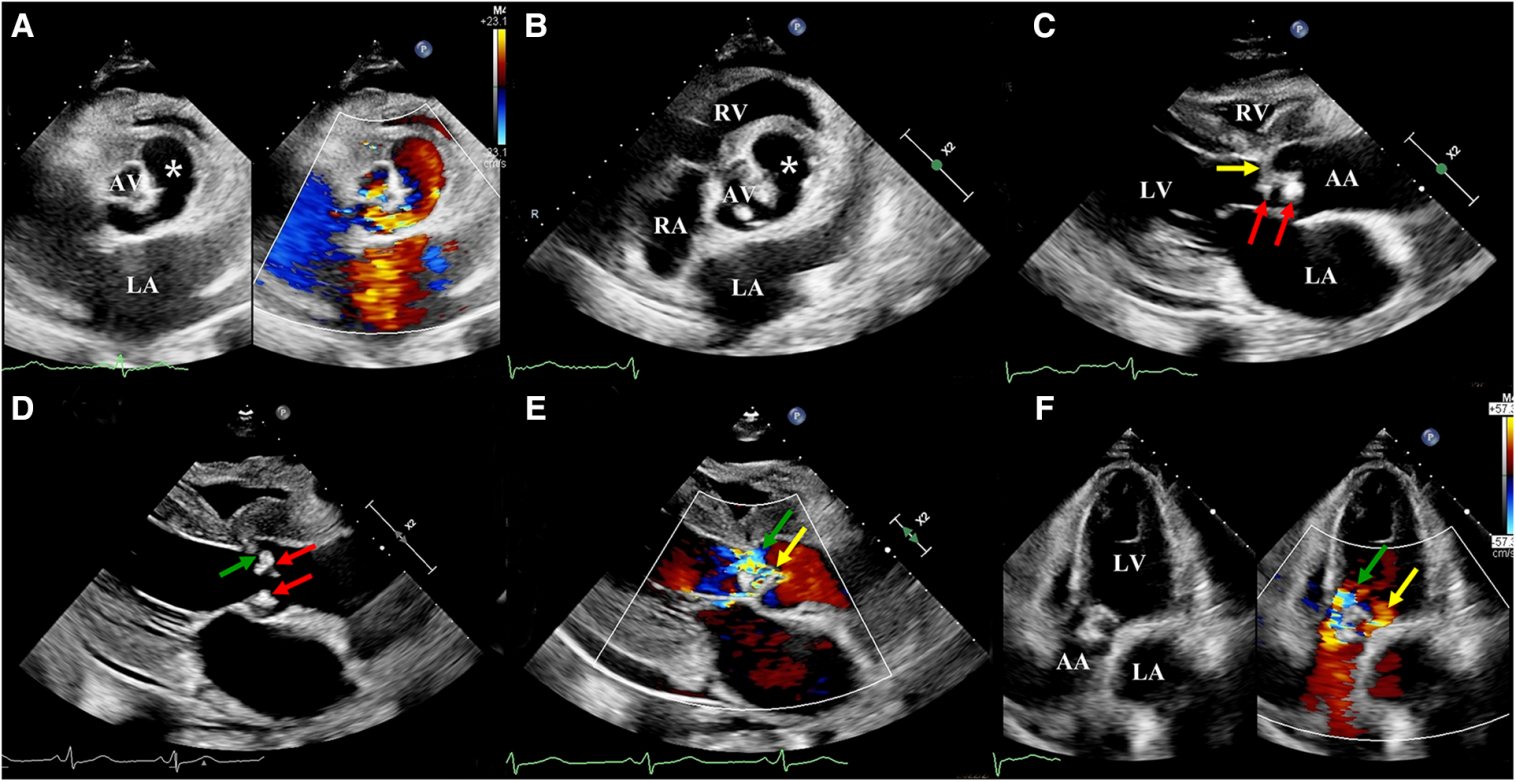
Two-dimensional transthoracic echocardiography: (A) A large sac-like aneurysm (white asterisk) located on the left front of the aortic root and communicating with it. (B) The number of aortic cusps could not be confirmed, as they were highly calcified and scattered with vegetation. (C) Aortic valve scattered with vegetations (red arrow) and local leaflets prolapsed (yellow arrow). (D) Aortic valve scattered with vegetation (red arrow) and local leaflet perforated (green arrow). (E) Parasternal long-axis view, color Doppler revealed two main aortic regurgitant jets, indicative of perforation (green arrow) and prolapsus (yellow arrow). (F) Apical five-chamber view, color Doppler revealed two main aortic regurgitant jets, indicative of perforation (green arrow) and prolapsus (yellow arrow). LA, left atrium; LV, left ventricle; RV, right ventricle; AA ascending aorta.
